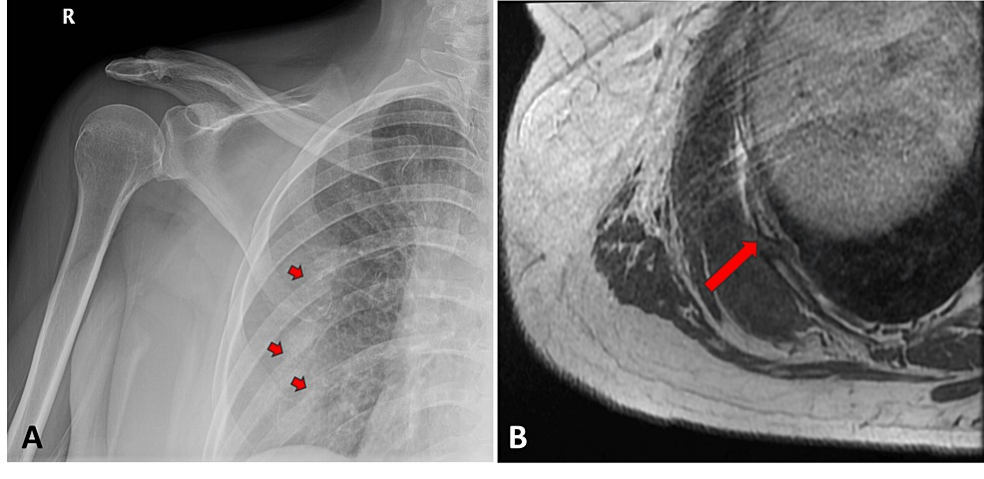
(A) Frontal view of the right shoulder radiograph showed multiple consecutive fractures of the right posterior sixth through eighth ribs (arrows). (B) Axial T1-weighted MRI of the chest demonstrated several subacute mildly displaced right posterolateral rib fractures (arrow) with callus formation and adjacent soft tissue edema.
